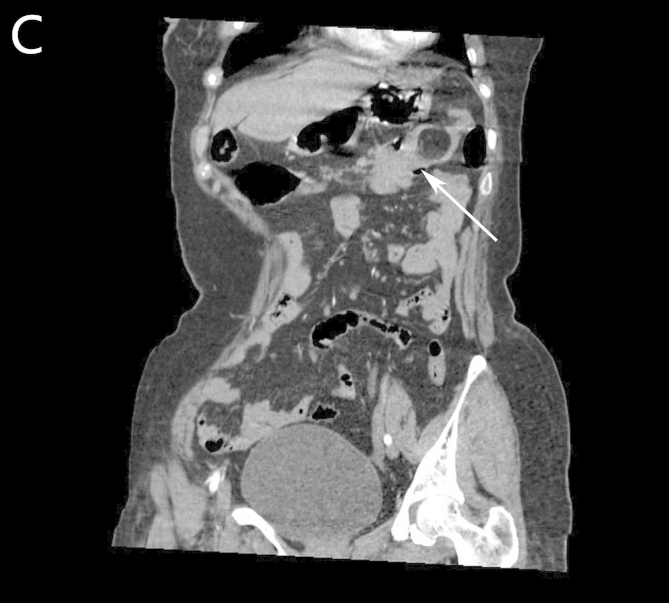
Contrast-enhanced computed tomography revealing (A) a well-defined fatty tissue mass of 4 cm in diameter (arrow) in the distal transverse colon proximal to the splenic flexure, with (B and C) intussusception (arrow) and local bowel-wall thickening.
